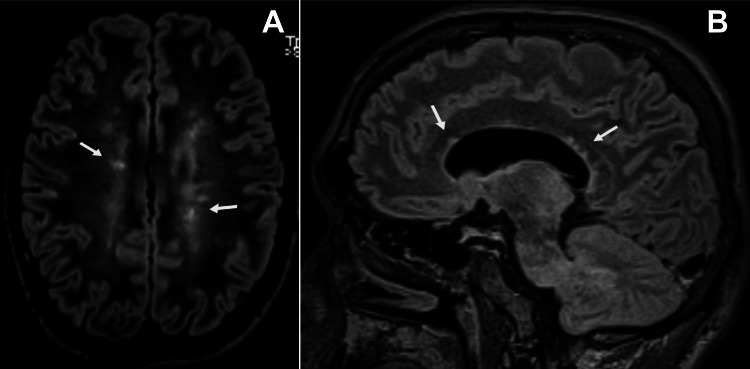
Brain magnetic resonance imaging, axial (A) and sagittal (B) view. (A) On an axial fluid-attenuated inversion recovery (FLAIR) sequence, several hyperintense lesions in the periventricular white matter can be depicted (arrows); they have an oval morphology, are perpendicular to the ventricles and involve the corpus callosum (this can be better appreciated on the sagittal view of a FLAIR sequence – B). They show no enhancement after administration of gadolinium (not shown).
